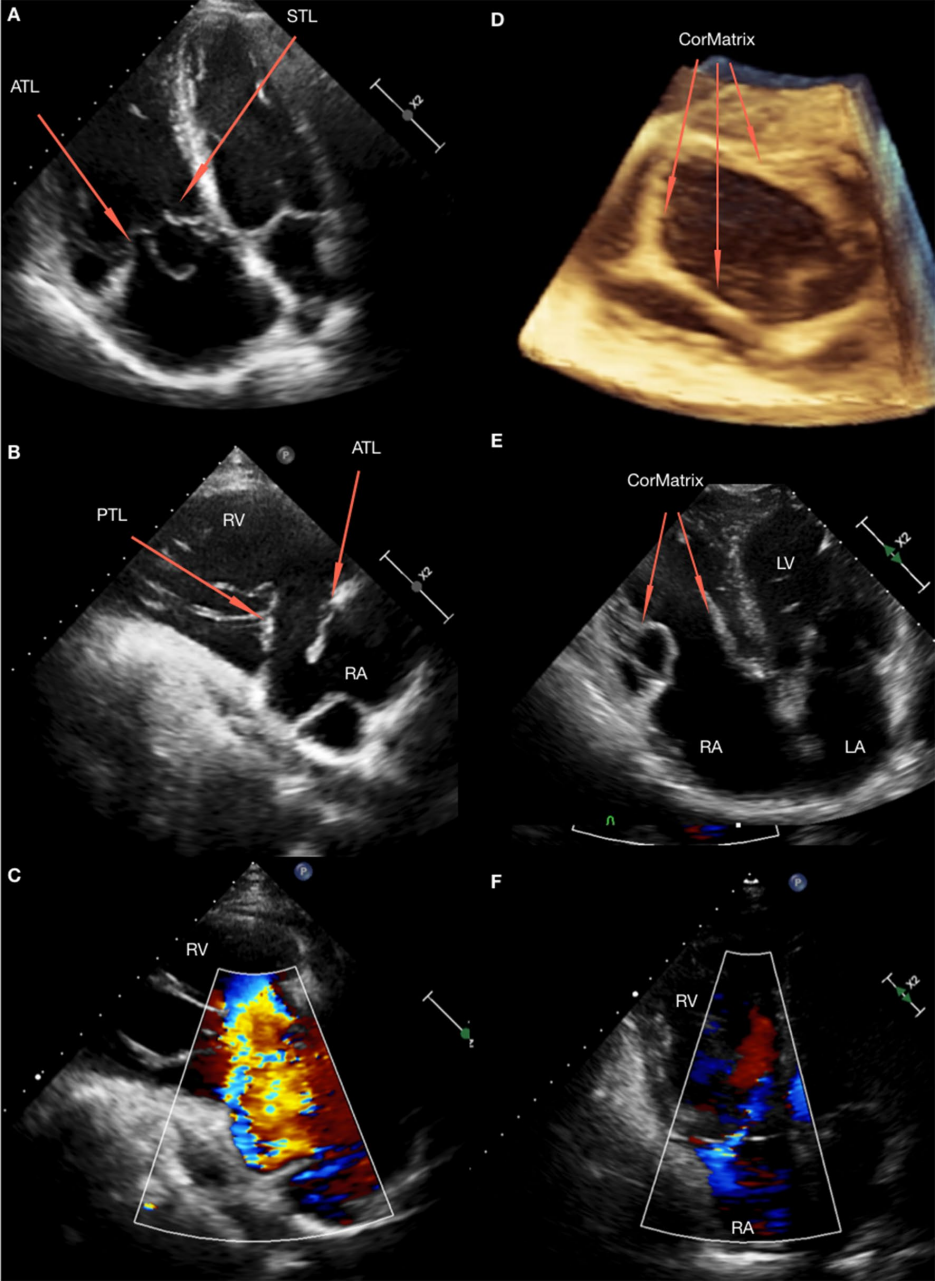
a, b Transthoracic echocardiography at admission- tricuspid valve leaflets, prolapse of the anterior leaflet, RV enlargement. c Transthoracic echocardiography at admission- severe tricuspid regurgitation. d 3D Intraoperative transesophageal echocardiography—tricuspid reconstruction using CorMatrix. e Follow-up transthoracic echocardiography—reduction of RV enlargement, proper valve function. f Follow-up transthoracic echocardiography—mild tricuspid regurgitation. *ATL* anterior tricuspid leaflet, *LA* left atrium, *LV* left ventricle, *PTL* posterior tricuspid leaflet, *RA* right atrium, *RV* right ventricle, *STL* septal tricuspid leaflet
